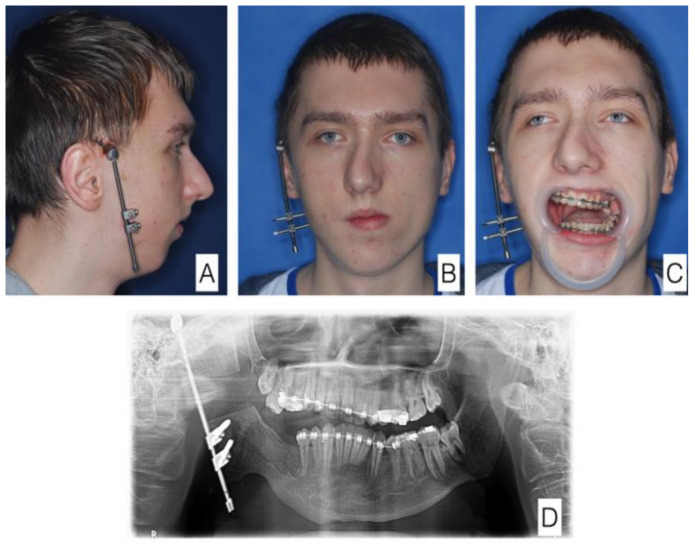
The same patient at a later stage of treatment. In the course of osseodistraction. (A) Lateral view of the face, (B) anterior view of the face, (C) anterior view of the face with mouth open—significant improvement visible. (D) orthopantomogram.
